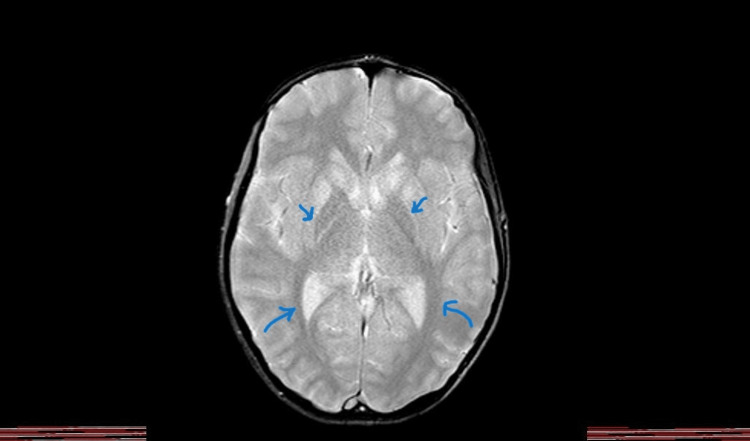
Brain MRI shows symmetric T2 and FLAIR hyperintense signals in the caudate nucleus and putamen
